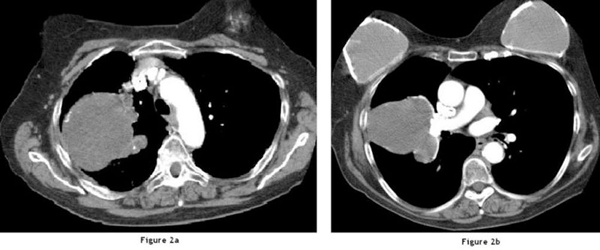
Computed tomography of the thorax at the level of aortic arch. (Left) This image shows a large cystic lesion in the right hemithorax with communication with the subsegmental dilated bronchi ('fingers in gloves' appearance). (Right) This image shows a section more caudally demonstrating enhancement within the medial wall of the lesion. Note is made of bilateral breast implants.
